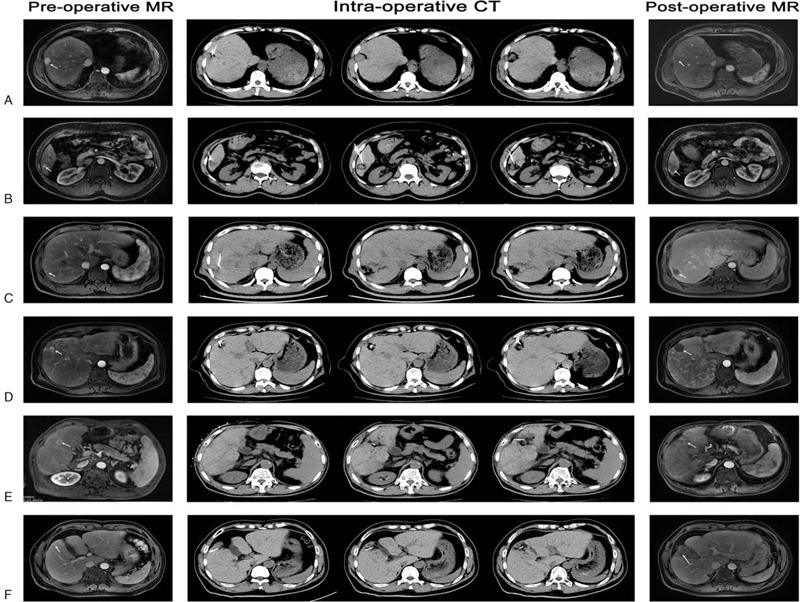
The preoperative MR (arterial phase), intraoperative CT (plane scanning) and postoperative month MR (arterial phase) of 6 HCC patients undergoing PPEI therapy. A, male, 38 years old, hepatocellular carcinoma in segment 8 of liver, tumor size: 3.1 × 2.6 × 2.3 cm, volume of ethanol injection: 55 ml. B, male, 52 years old, hepatocellular carcinoma in segment 6 of liver, tumor size: 1.6 × 1.4 × 1.2 cm, volume of ethanol injection: 29 ml. C, male, 40 years old, hepatocellular carcinoma in segment 7 of liver, tumor size: 0.9 × 0.8 × 0.7 cm, volume of ethanol injection: 20 ml. D, male, 63 years old, hepatocellular carcinoma in segment 5 of liver, tumor size: 1.7 × 1.6 × 1.4 cm, volume of ethanol injection: 32 ml. E, male, 61 years old, hepatocellular carcinoma in segment 6 of liver, tumor size: 2.4 × 2.1 × 1.7 cm, volume of ethanol injection: 43 ml. F, female, 50 years old, hepatocellular carcinoma in segment 5 of liver, tumor size: 2.1 × 1.4 × 1.2 cm, volume of ethanol injection: 33 ml. CT = computed tomography, HCC = hepatocellular carcinoma, MR = magnetic resonance, PPEI = percutaneous peritumor ethanol injection.
